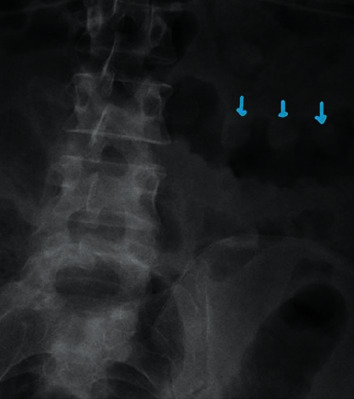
Abdomen X-ray showing thumbprinting sign.
